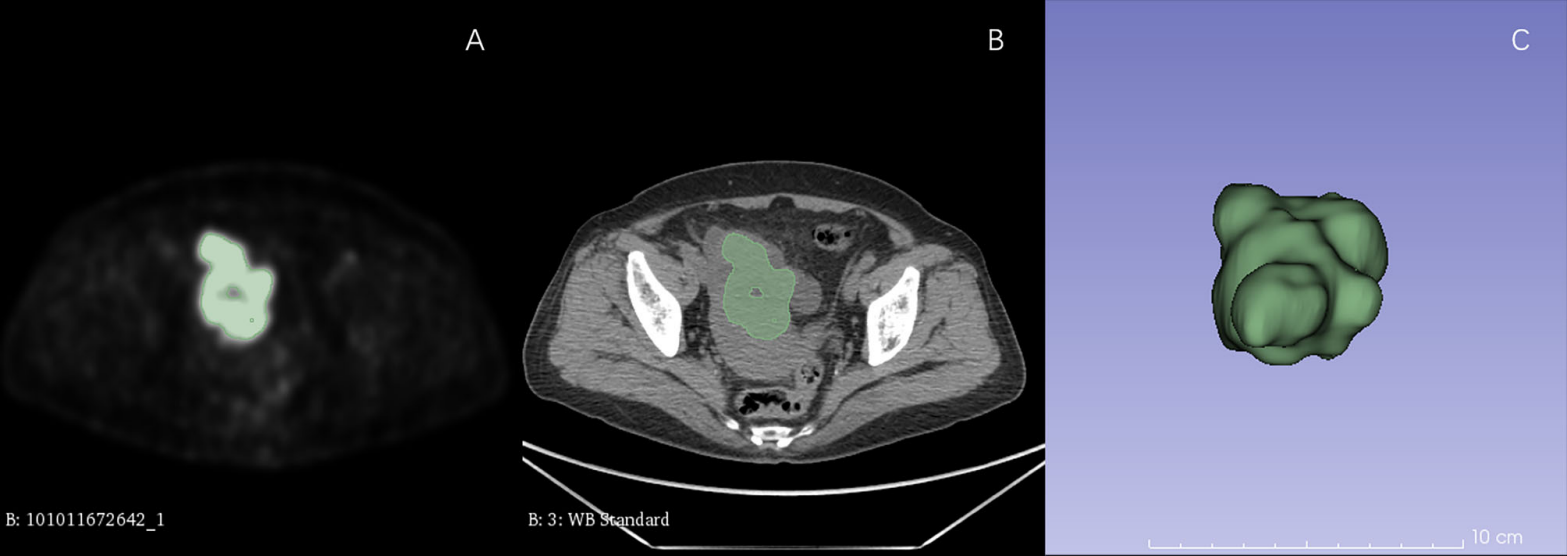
ROI delineation in PET and CT images. Plots (A, B) showed ROI delineation in PET and CT images. Plots (C) showed the 3D view.
